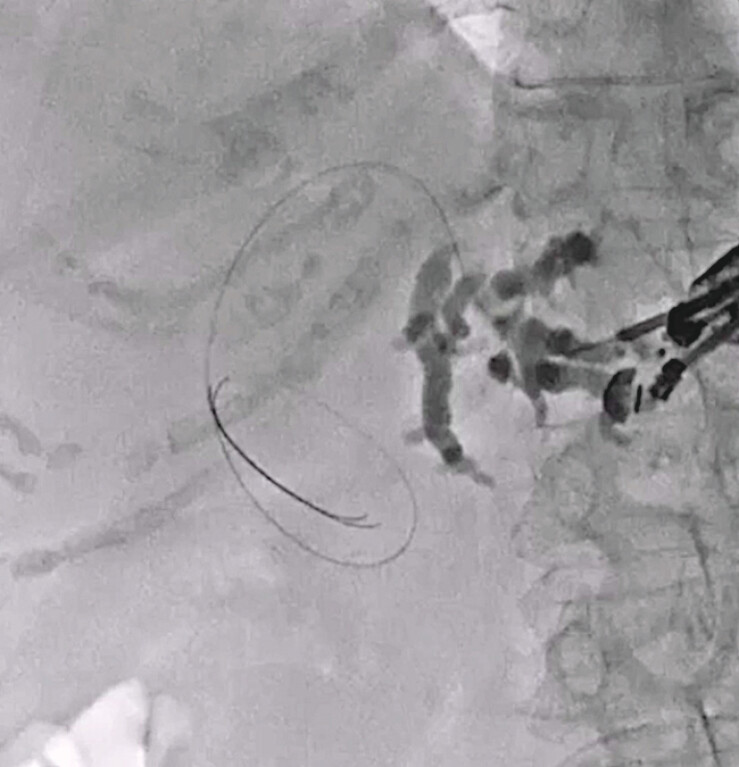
A guidewire is deployed under endoscopic ultrasound guidance.
